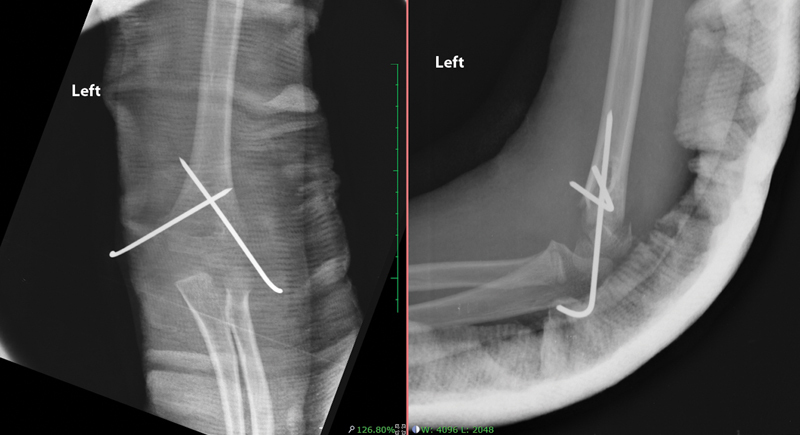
Anteroposterior (AP) and lateral radiographs of the distal humerus performed immediately after surgery, demonstrating unsatisfactory reduction, as the anterior humeral line does not touch the capitellum. In the AP radiograph, the ulnar spine wire does not appear to fixate the distal fragment.
